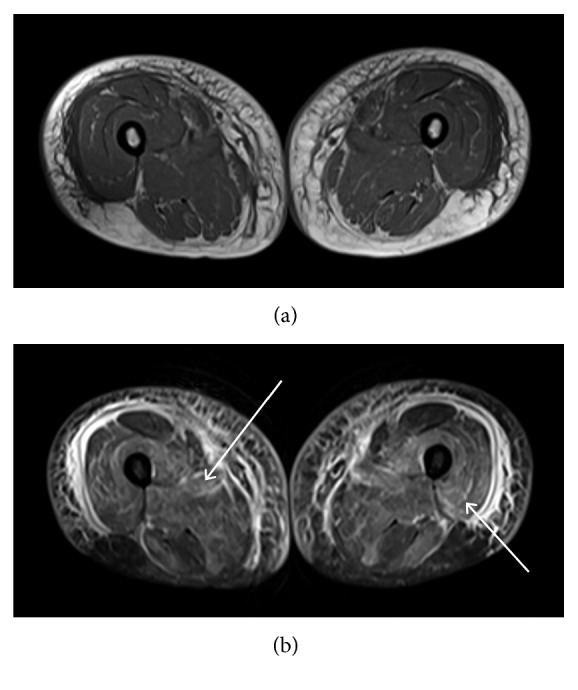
(a) T1-weighted and (b) short tau inversion recovery (STIR) sequences showing edema (hyperintense areas on STIR, white arrows) in the proximal thigh muscles, characteristic of an inflammatory myositis.
